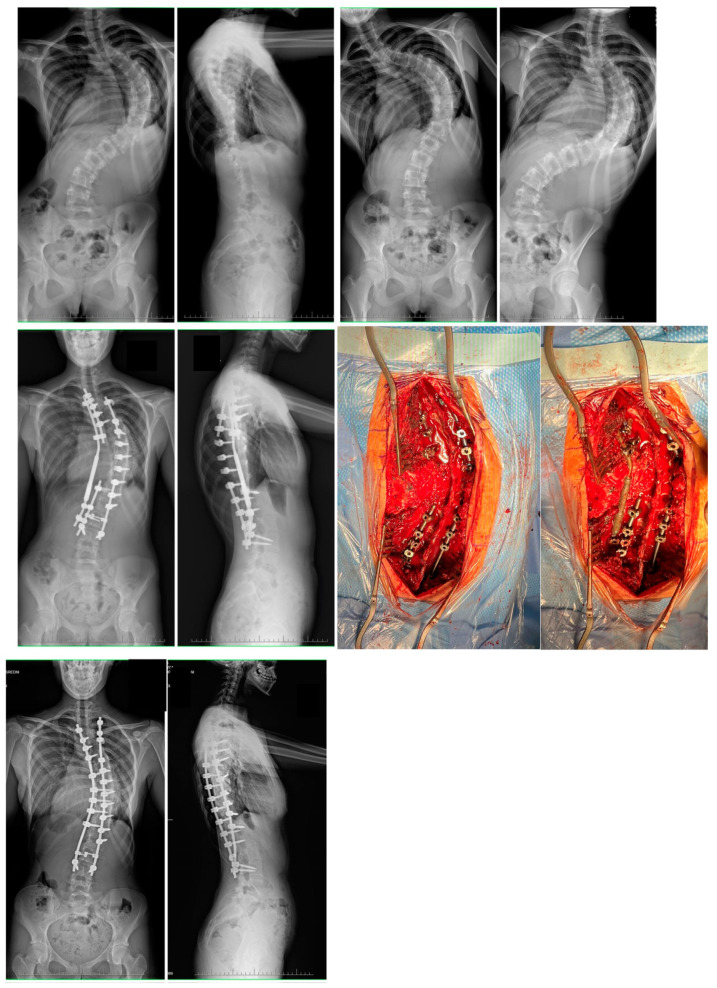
A 16-year-old girl with severe adolescent idiopathic scoliosis treated with staged surgery using MCGR as temporary internal distraction followed by final correction with double Co-chr 6.0 rods. Pre- and postoperative radiographs during observation period and intraoperative pictures shown using MCGR as a temporary internal distraction device.
